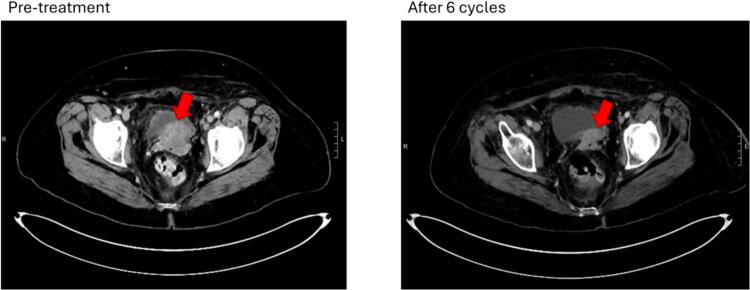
Radiographic response in patient with four prior lines of therapy. This patient had previously received carboplatin and paclitaxel, followed by weekly paclitaxel, lenvatinib and pembrolizumab, and single agent bevacizumab. Red arrow demonstrates significant decrease in volume of a vaginal cuff tumor.
